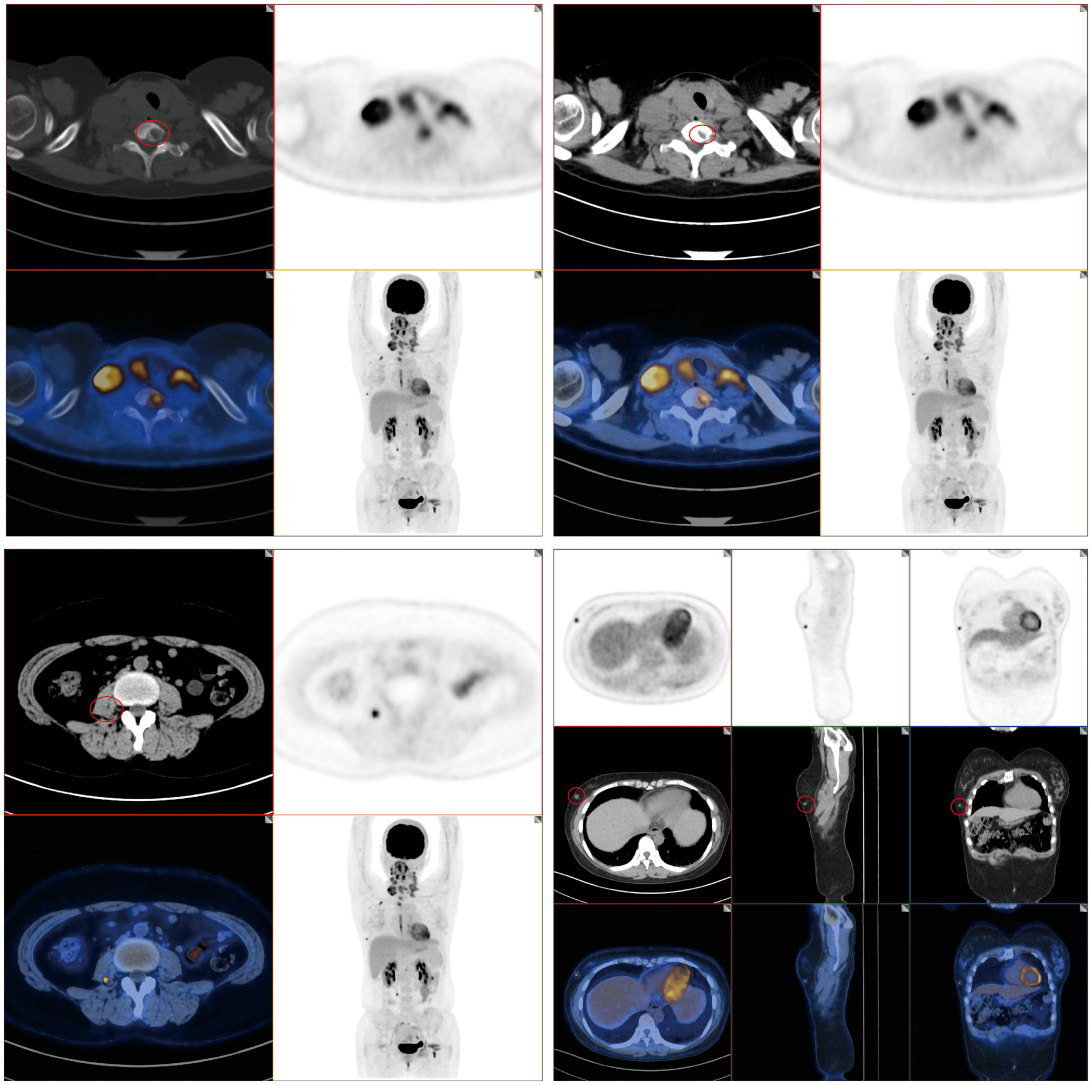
PET-CT results of patients. PET-CT imaging revealed multiple, slightly hypoechoic nodules within the bilateral thyroid lobes, suggesting the possibility of lymph node metastasis in the bilateral neck, supraclavicular region, and mediastinum. Furthermore, soft tissue density nodules were discernible in the outer lower quadrant of the right breast. Additionally, a nodular abnormal radioactive concentration shadow was observed in the right psoas major muscle; bone destruction was evident in the right second rib and seventh cervical vertebra.
